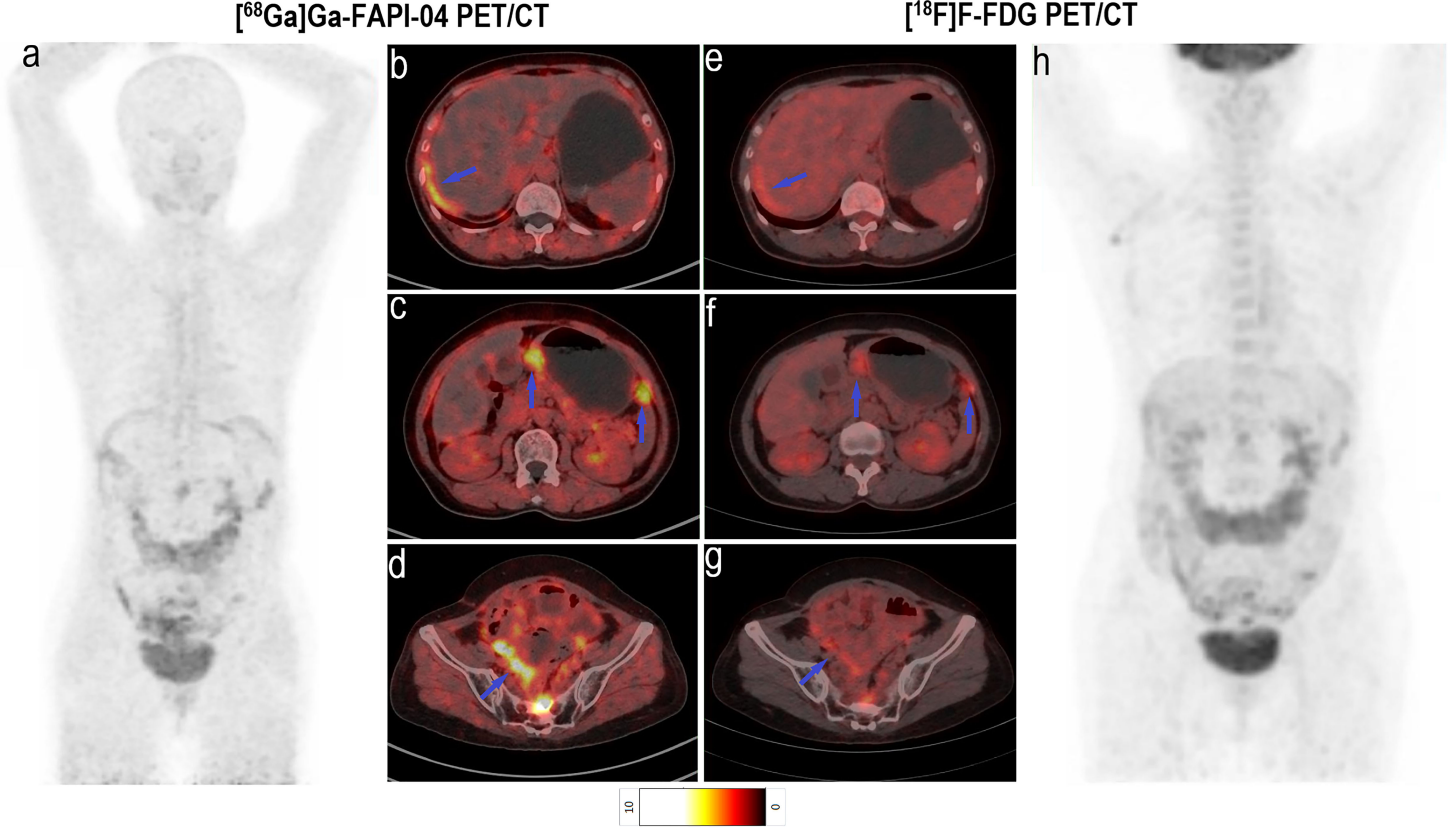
Images of a 60-year-old woman with colon cancer and metastatic peritoneal carcinoma for restaging after treatment. (A) Mean intensity projection images of [^68^Ga]Ga-FAPI-04 PET/CT scans. (B–D) Axial fused images of metastatic peritoneal carcinomas at different body levels in [^68^Ga]Ga-FAPI-04 PET/CT. (E–G) Axial fused images of metastatic peritoneal carcinomas at different body levels in [^18^F]F-FDG PET/CT. (H-h) Mean intensity projection images of [^18^F]F-FDG PET/CT scan. [^68^Ga]Ga-FAPI-04 uptake (blue arrows in B–D) was higher than the [^18^F]F-FDG uptake (blue arrows in E–G) in peritoneal carcinomas, and SUV_max_ values for [^68^Ga]Ga-FAPI-04 and [^18^F]F-FDG PET/CT were 4.6–8.8 and 3.1–3.5, respectively.
